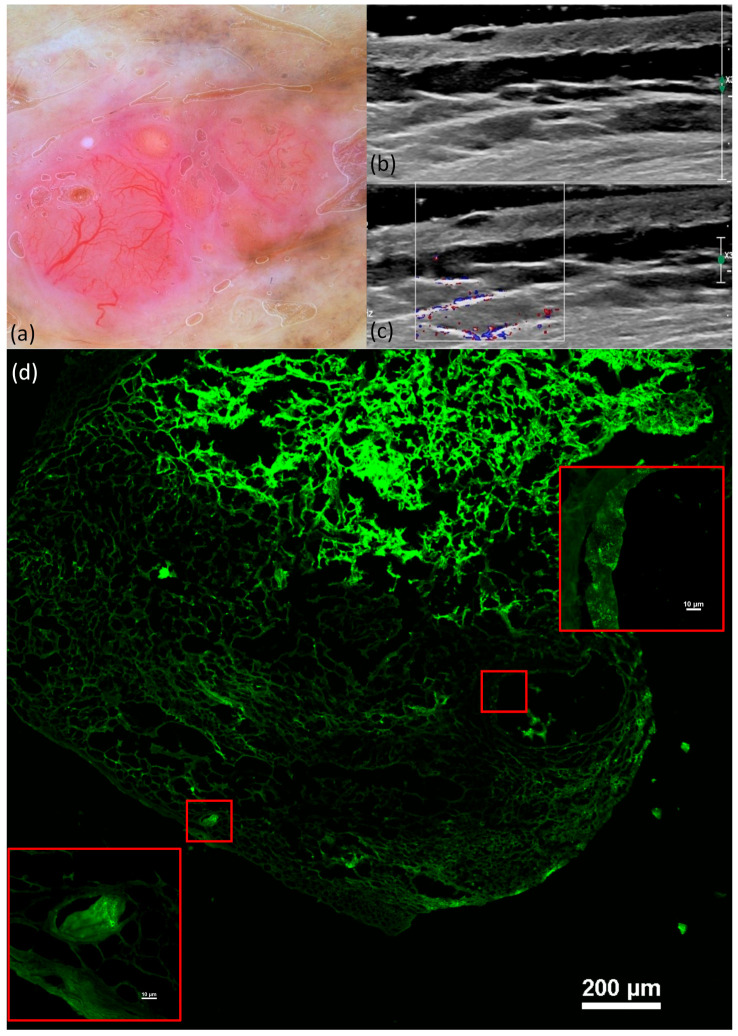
Nodular BCC. Dermoscopy shows arborized vessels and shiny white-red structureless areas (a). US shows a hypoechoic lesion, imprecisely delimited, that reaches the level of the reticular dermis (b) without a Doppler signal (c). FCM shows well-defined tumor islands and a palisading, clefting, and “cauliflower-like” appearance (d).
